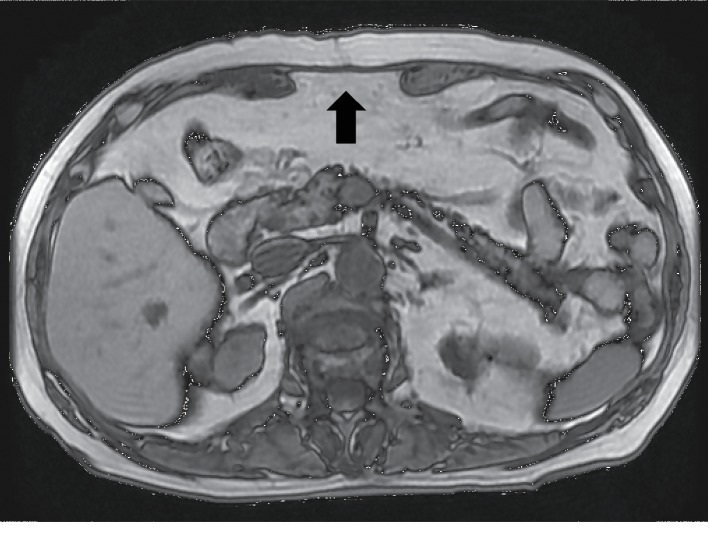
Magnetic resonance imaging reveals the implanted fascia lata graft (arrow).
